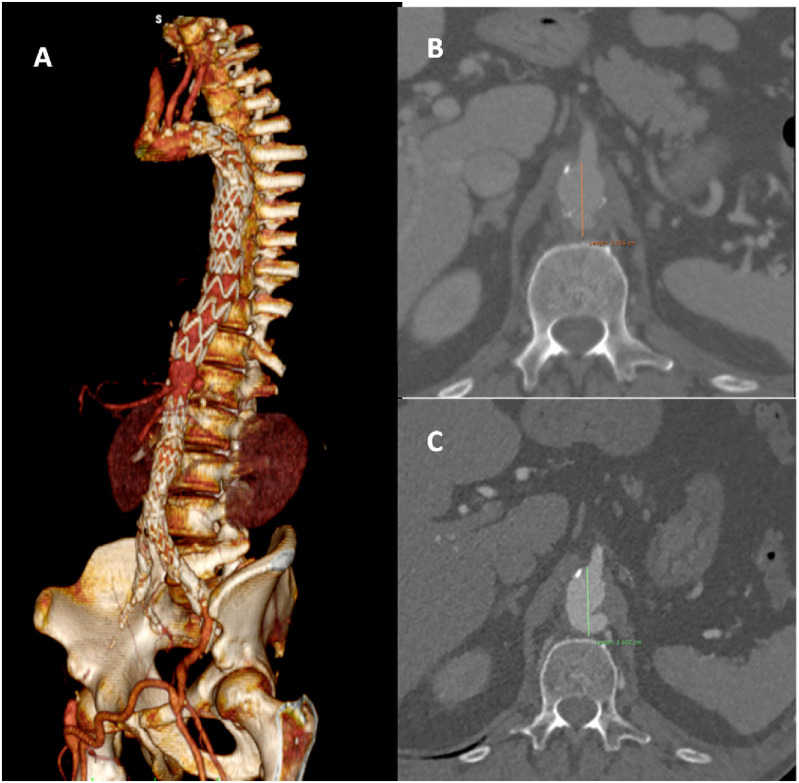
Fig. 2 Three-dimensional computed tomography (CT) reconstruction in lateral view showing the correct deployment of both thoracic and abdominal aorta, the femoro–femoral crossover bypass patency, and the residual posterior penetrating aortic ulcer (PAU) of the visceral aorta (A). Visceral aortic PAU of 30 mm at the first CT scan (B), increased to 36 mm at the last CT scan (C).
